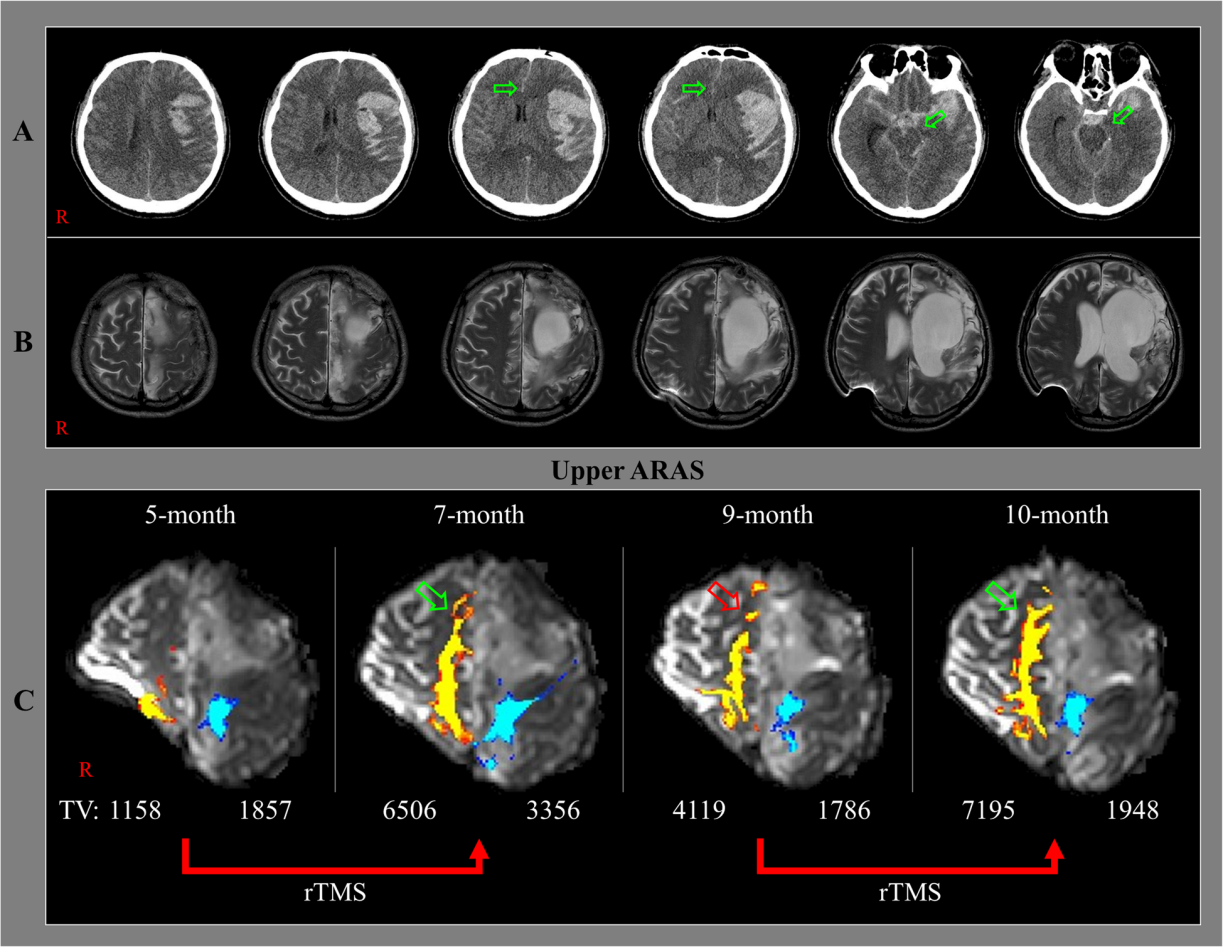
a Brain computed tomography images at onset show subfalcine and transtentorial herniations (arrows). b Brain magnetic resonance images at 5 months after onset show leukomalactic lesions in the left fronto-parieto-temporal lobes. c Results of diffusion tensor tractography (DTT) of the upper ascending reticular activating system (ARAS) of the patient. On the 7-month DTT, the neural tract of the right prefrontal lobe is increased (green arrow) compared to that on the 5-month DTT. However, the same neural tract is decreased (red arrow) on the 9-month DTT, but it is again increased (green arrow) on the 10-month DTT. ARAS: ascending reticular activating system, TV: tract volume, rTMS: repetitive transcranial magnetic stimulation
